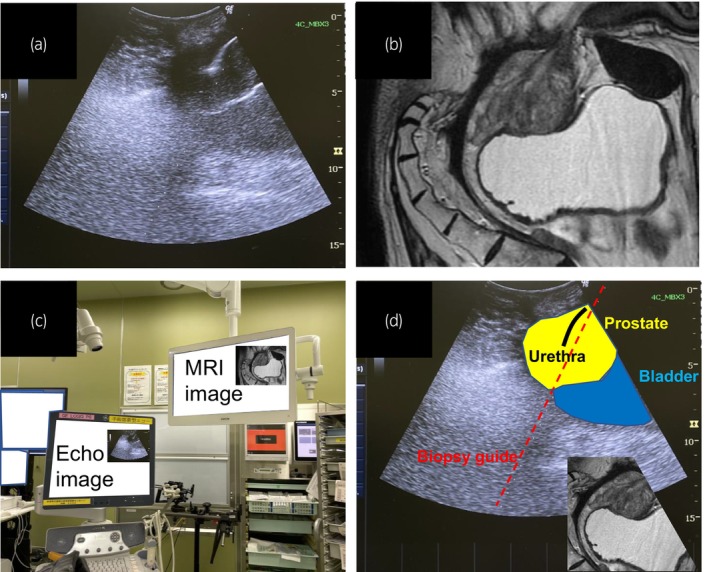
Cognitive MRI‐TPUS fusion biopsy. (a) TPUS scanning. The probe was placed vertically along the body to create a sagittal view. (b) Sagittal MRI of the prostate was flipped upside down. (c) The arrangement of devices to perform cognitive MRI‐TPUS fusion prostate biopsy. (d) Cognitive MRI‐TPUS fusion image.
